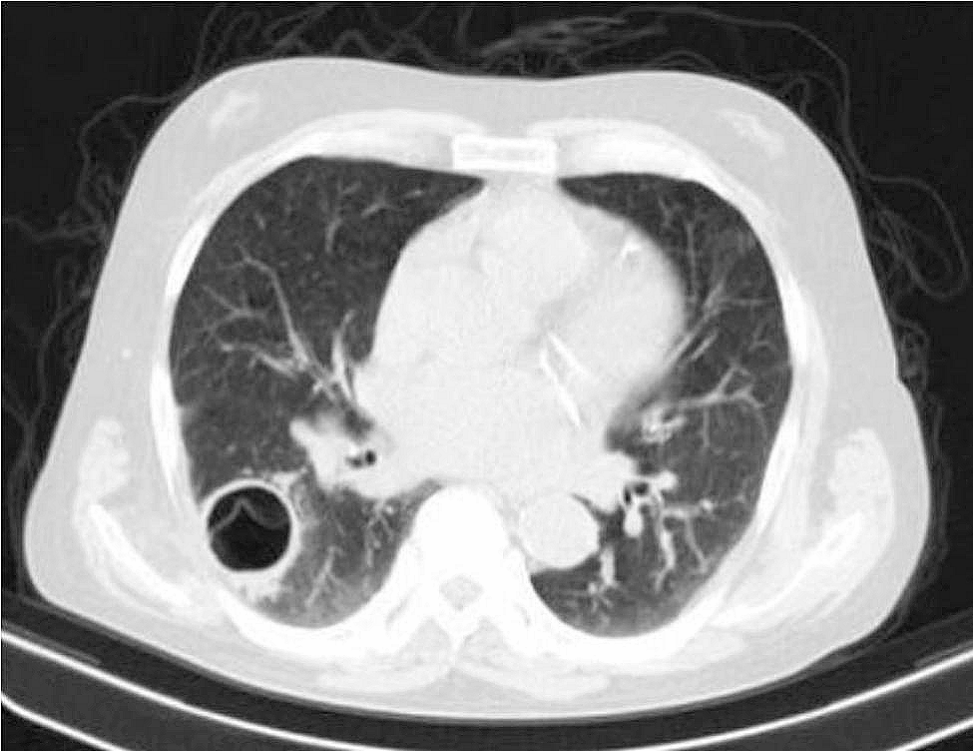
Lung CT scan of a 40-year-old man with concomitant hydatid cyst. Two cavitary lesions with internal floating membrane with peripheral alveolar opacification
